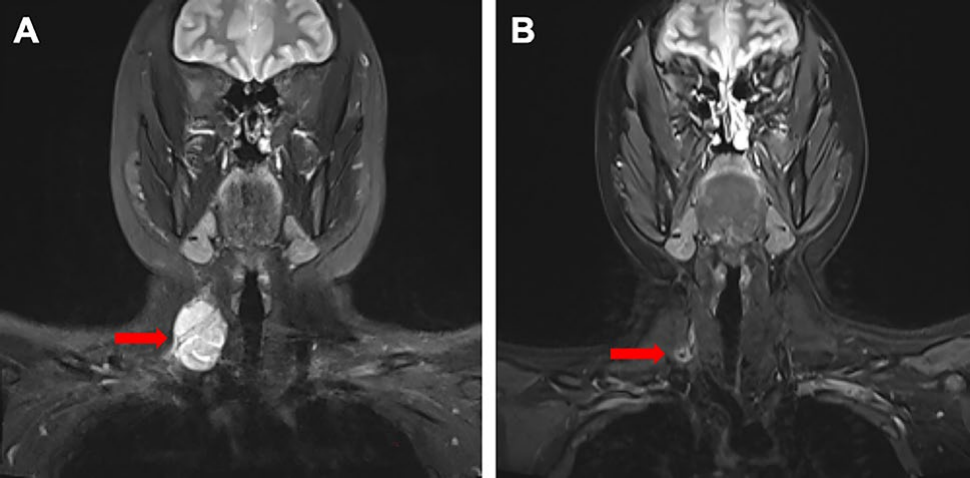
Magnetic resonance imaging scan of a synovial sarcoma patient before neoadjuvant therapy (A) and before resection (B). The red arrow indicates the primary lesion
